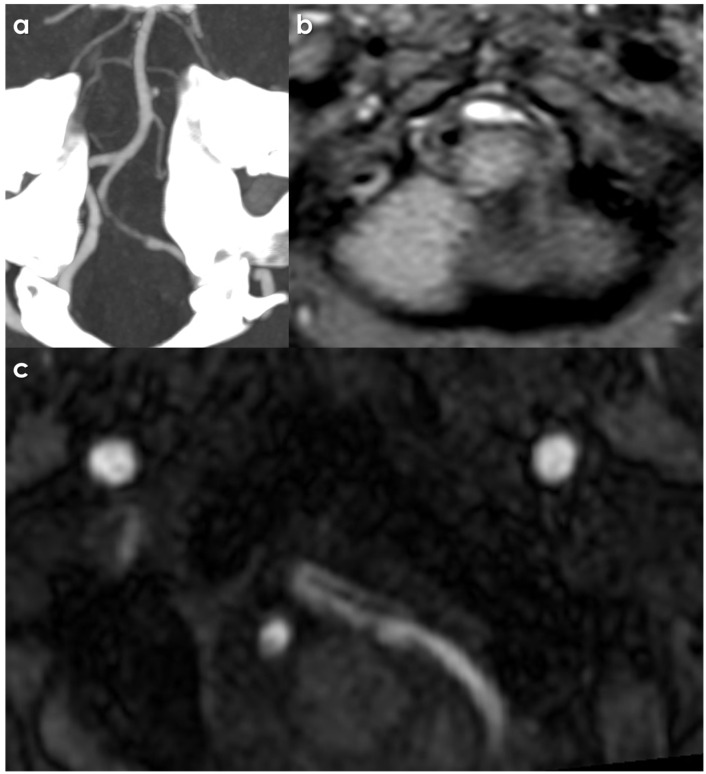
Intracranial left VAD. The CTA panel (a) shows a long-tapered stenosis, corresponding with the flow in the true lumen, as outlined by the presence of a mural hematoma and hyperintense T1 Dixon MRI panel (b) with an intimal flap (panel (c), MRA source image).
